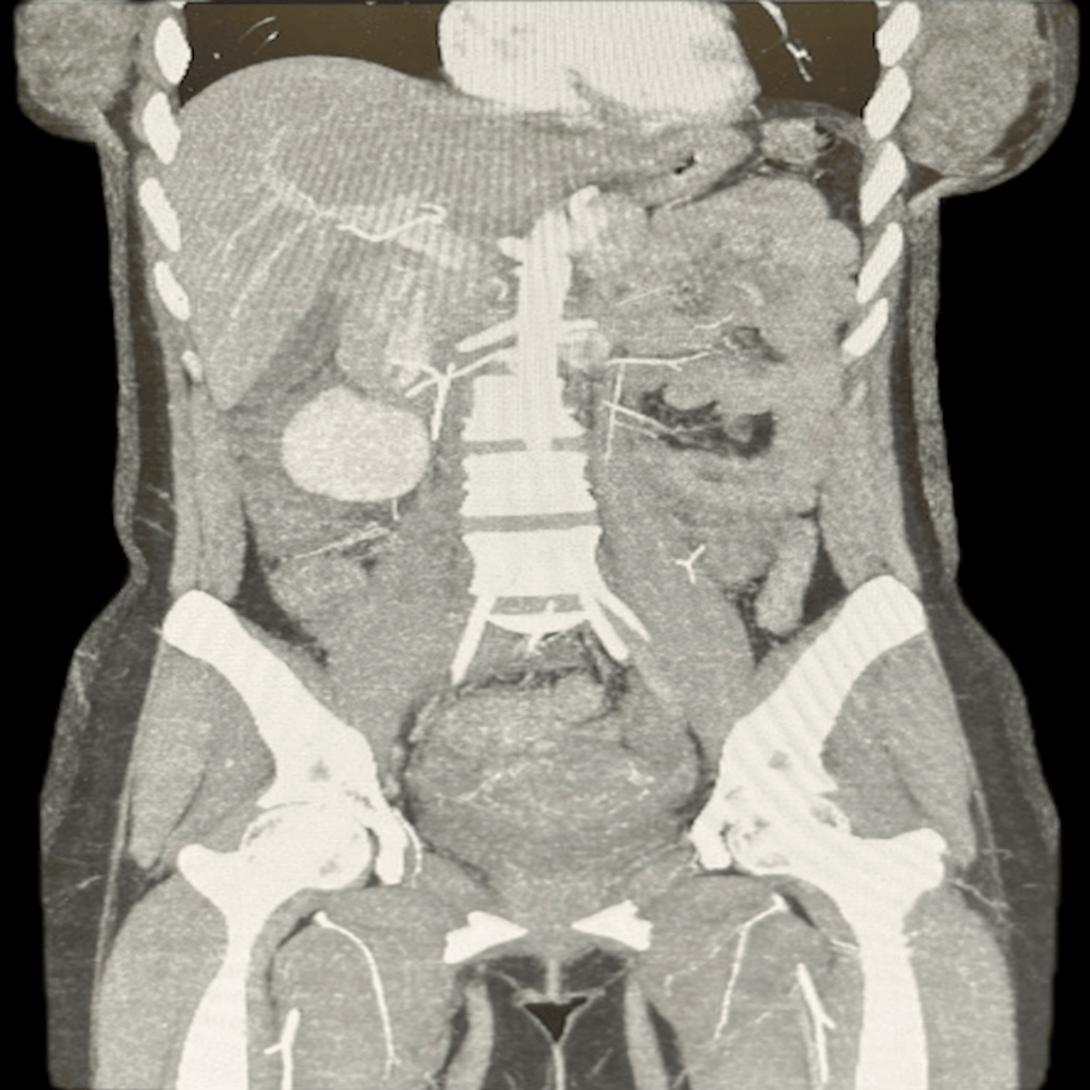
A repeat CT scan taken seven days from the last procedure found no signs of extravasation and showed decreasing fluid collection.
